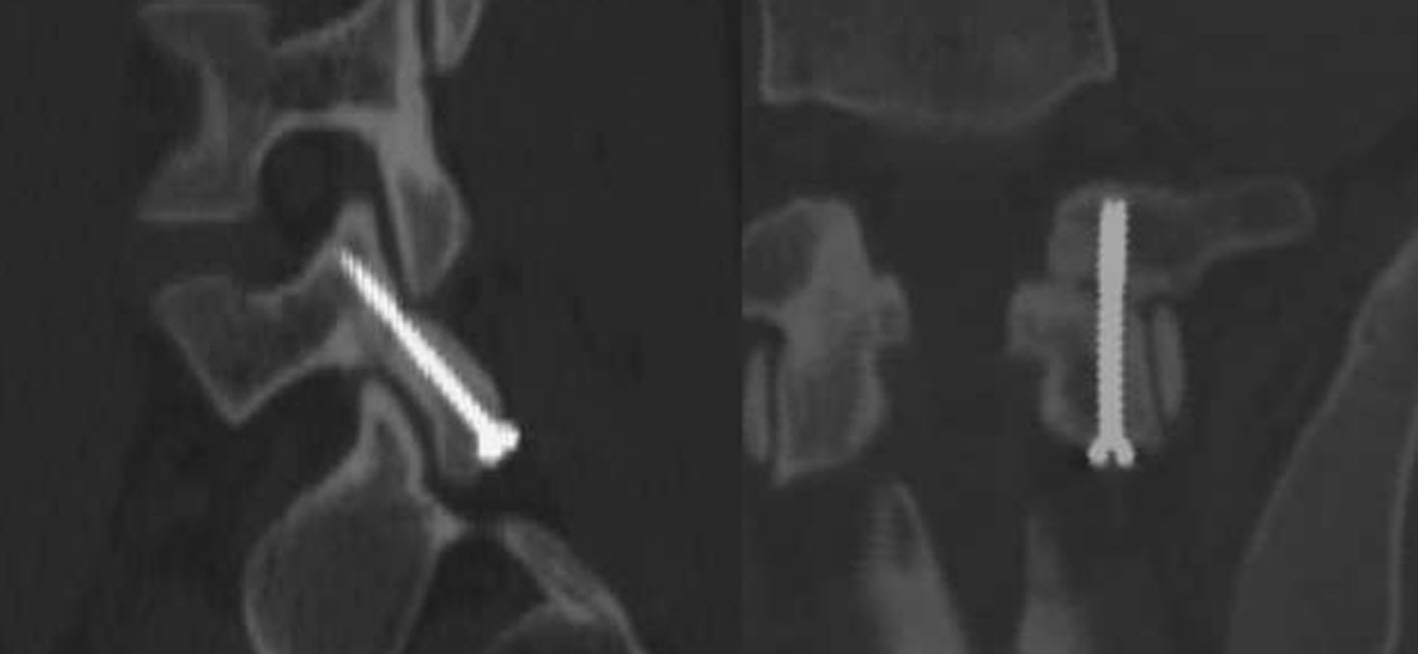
Postoperative CT scan in sagittal (*left figure*) and coronal (*right figure*) plane
